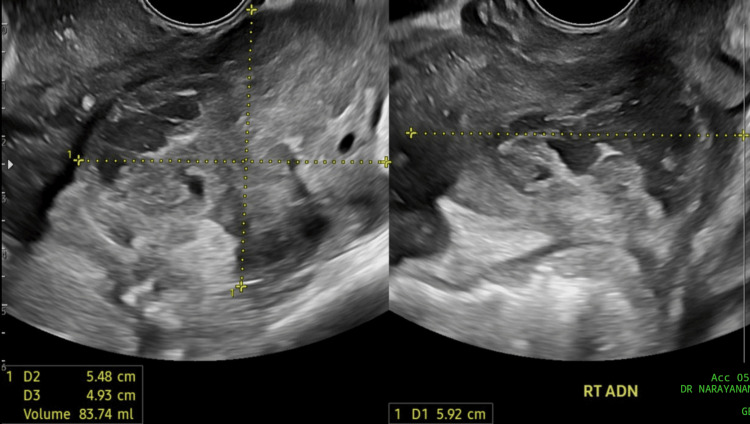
Postoperative transvaginal ultrasound Transvaginal ultrasound shows a relatively well defined heterogeneous mass lesion in the right adnexa.
